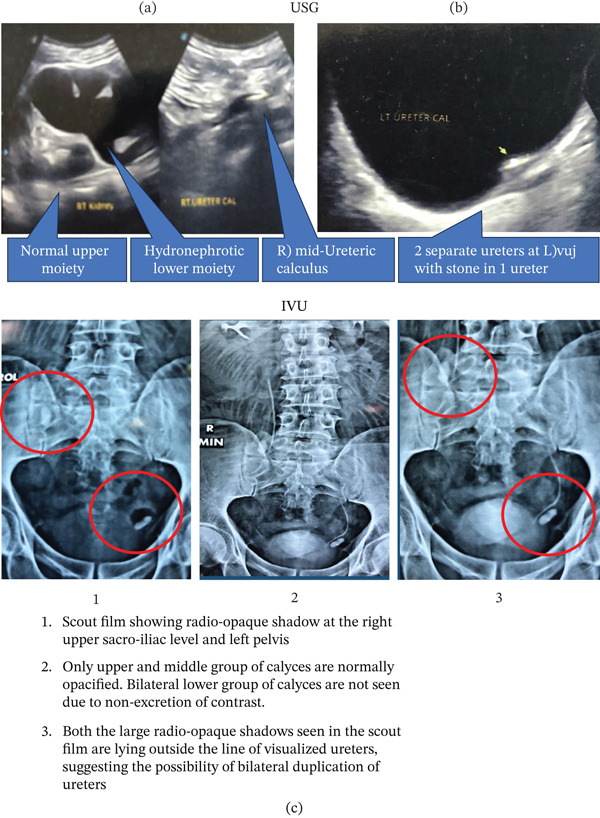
(a) The ultrasonography of the right kidney and mid‐ureter showing normal upper moiety and hydronephrotic lower moiety due to a large mid‐ureteric calculus. (b) The ultrasonography of the urinary bladder showing two ureteric openings at the left vesicoureteric junction and a calculus in one ureter. (c) The scout film showing radio‐opaque shadow at the right upper sacroiliac level and left pelvis. Only the upper and middle group of calyces are normally opacified. Bilateral lower group of calyces are not seen due to nonexcretion of contrast. Both the large radio‐opaque shadows seen in the scout film are lying outside the line of visualized ureters, suggesting the possibility of bilateral duplication of ureters.
